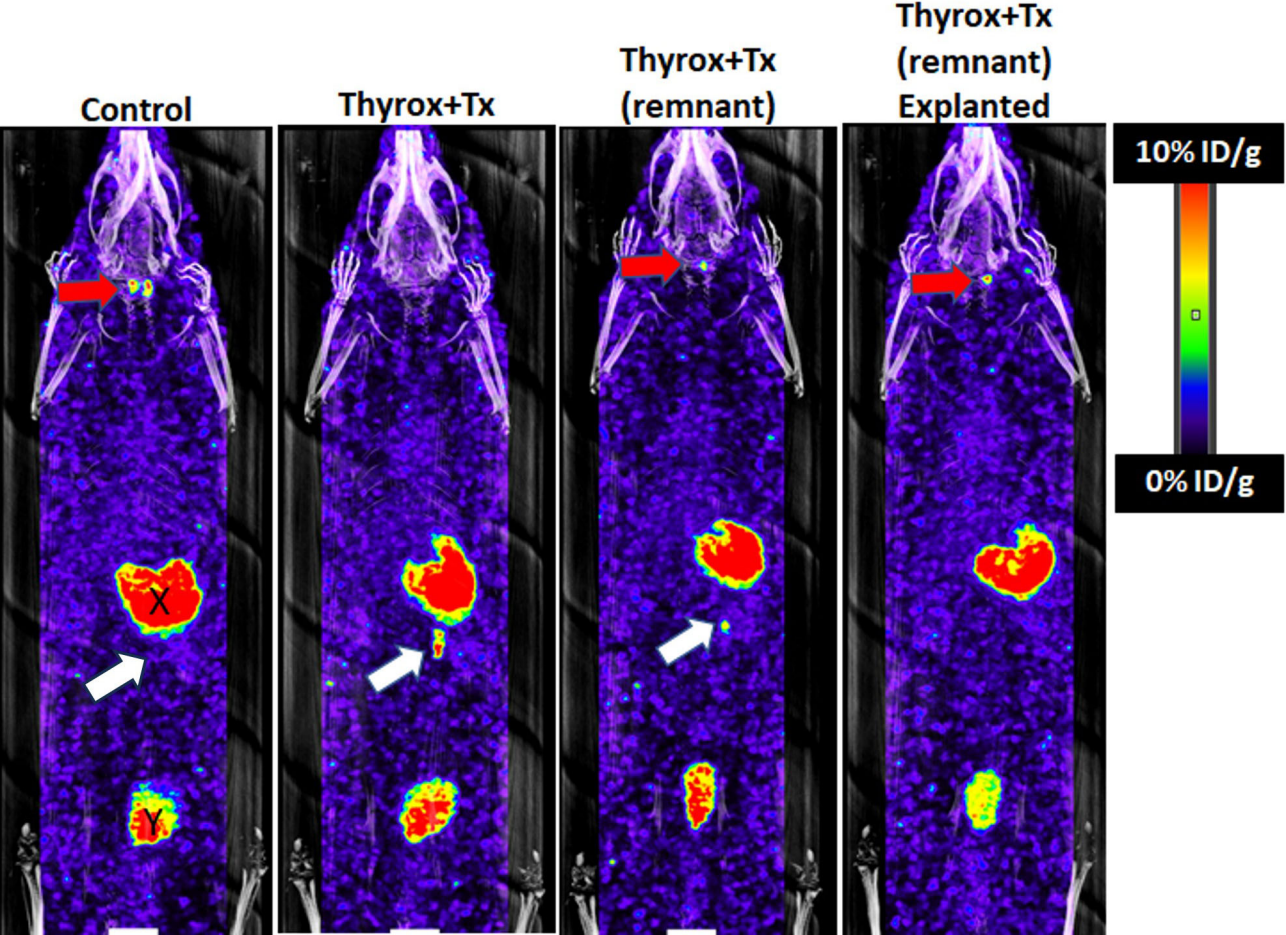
Whole-body scintigraphy comparing isotope uptake. The control shows typical thyroid uptake (red arrow) and no uptake in the Cell Pouch (device only, white arrow) implant site. In Thyrox+Tx subjects, reduced or absent uptake in the thyroid bed indicates total thyroidectomy or residual lobe function, respectively. Significant uptake in the Cell Pouch area (white arrows) is evident, which ceases after the pouch’s removal (explant), signifying the graft’s confinement to the device. “X” and “Y” mark the epigastric and bladder, known for high technetium uptake, respectively.
